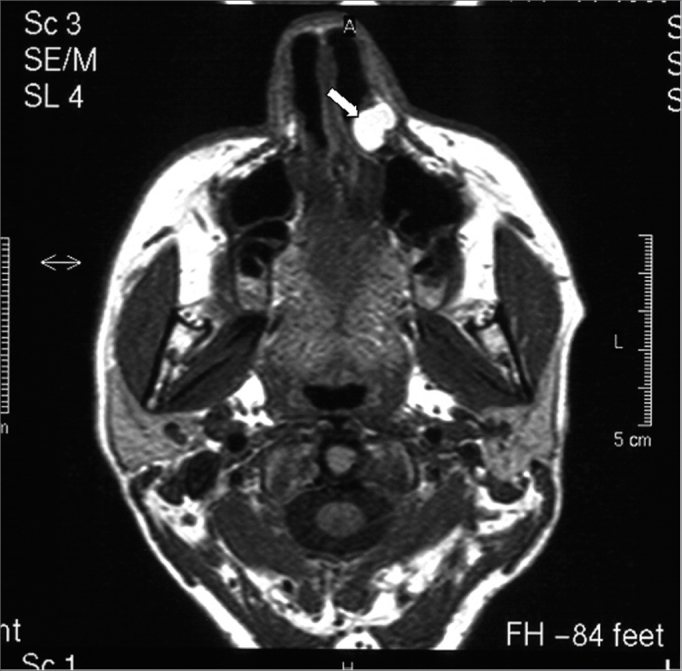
A-) T1-weighted MRI image (axial section). Note a hyperintense area in the left nose that suggests an elevated protein content.
